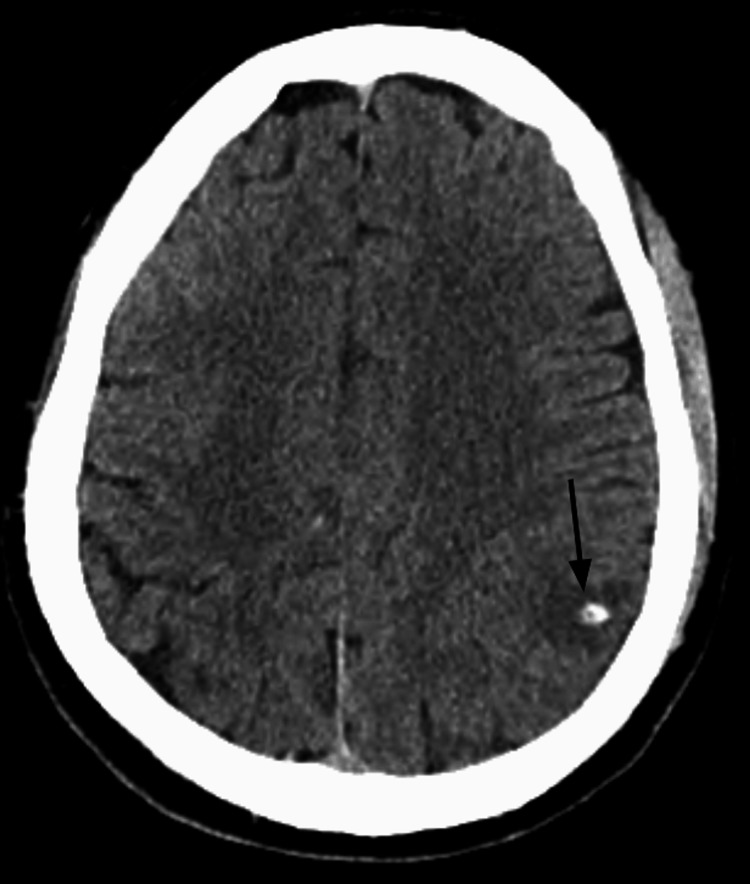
CT head reveals a cystic lesion in the mesial left parietal lobe with peripheral calcification, indicative of a scolex (vesicular stage)
